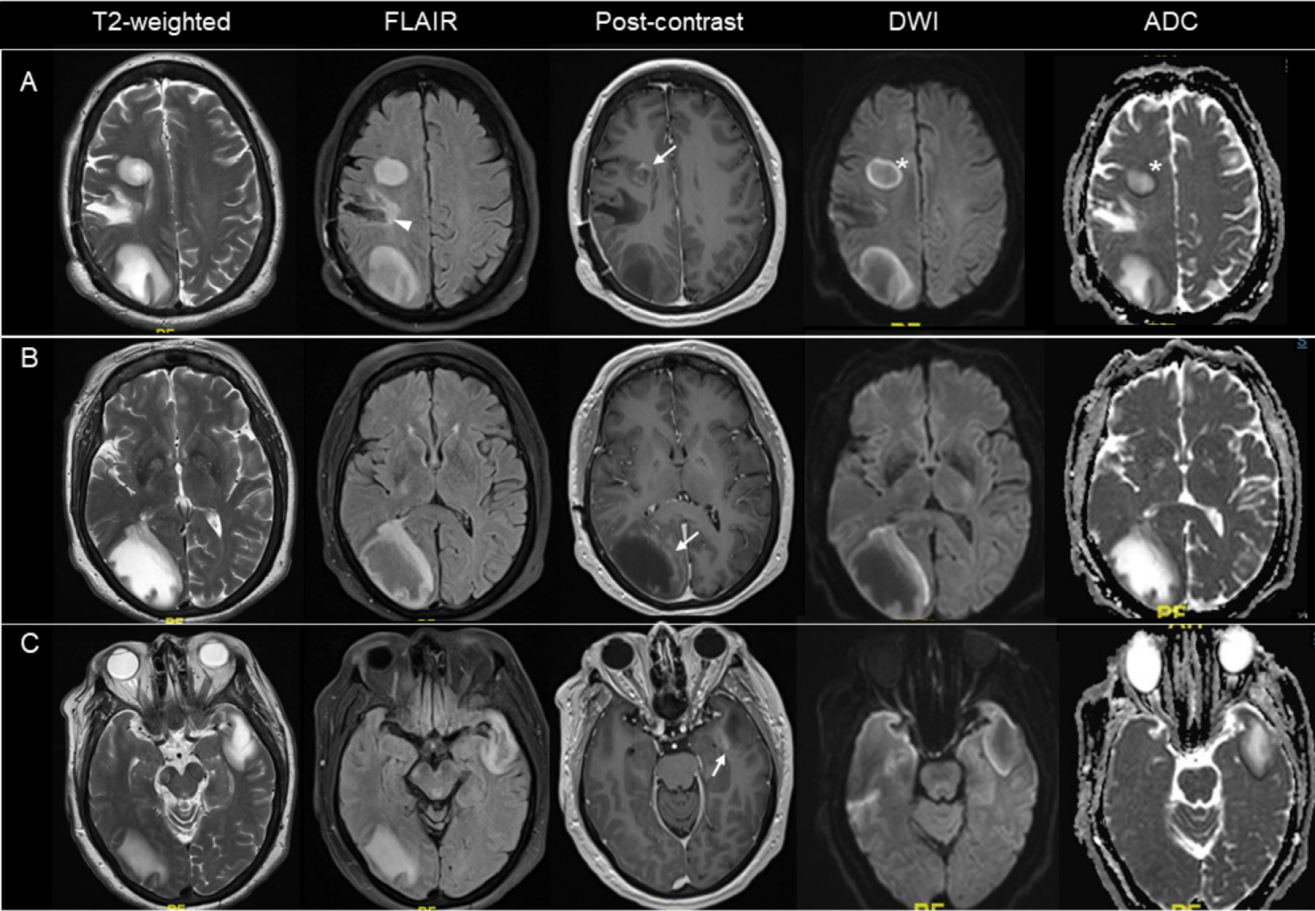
Brain MRI, 5 months following initial hospitalization, demonstrating interval development of multiple tumefactive demyelinating lesions. Axial brain MRI with T2-weighted, fluid inversion recovery imaging, postcontrast, diffusion weighted and apparent diffusion coefficient images (labeled columns) showing interval decrease in size of the previous right frontoparietal lesion (A, arrowhead) but development of three hyperintense lesions involving the right frontal centrum semiovale, right occipital lobe and left anterior temporal lobe which have associated edema but exhibit relatively little mass effect. All lesions show partial rim-enhancement on postcontrast images (A–C, arrows). The lesions show peripheral, but not central, restricted diffusion (A, stars).
